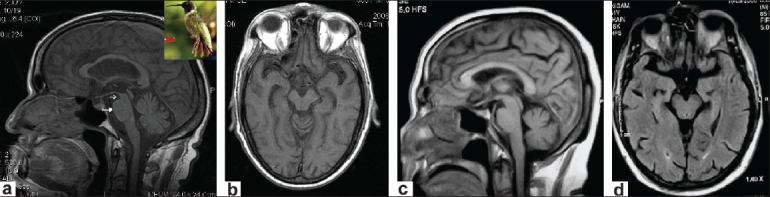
(a) Midsagittal T1W MRI brain showing atrophy of the midbrain tegmentum (open arrow) with a relatively preserved pons (closed arrow), resembling the head and body, respectively, of a hummingbird (inset). (b) Midbrain tegmentum atrophy is evident in axial T1W MRI of the brain. (c and d) Midsagittal and axial T1W MRI of the brain of a normal age and sex mathced control
